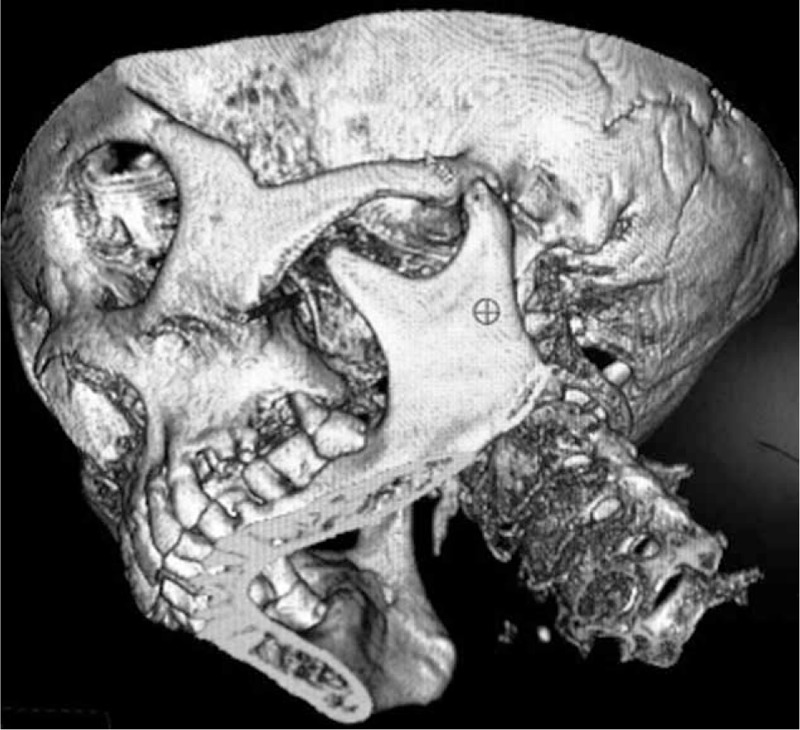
A 3D CT reconstruction showing the trajectory of the needle after insertion. CT = computed tomography.
